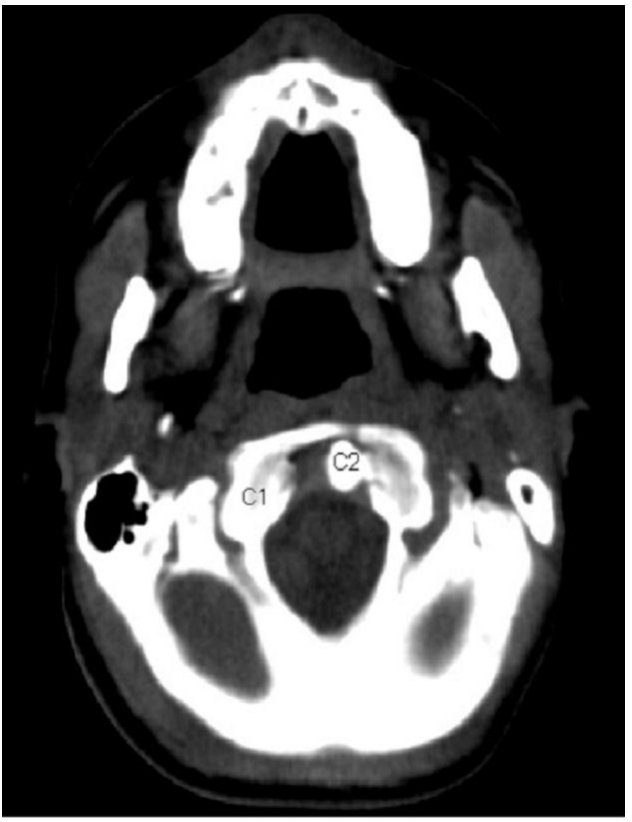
Axial CT image demonstrated AARF; the right lateral mass of the atlas C1 lies anterior to the articular surface of C2.
